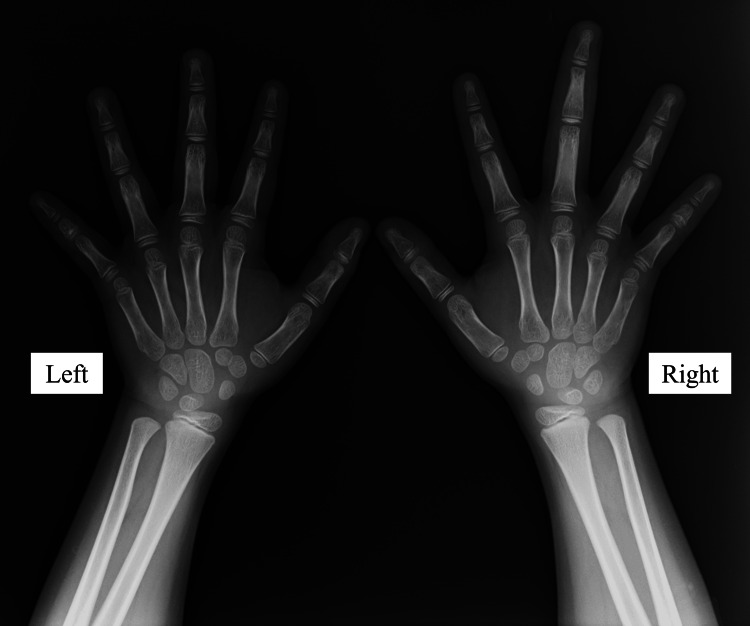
Radiographic images of the patient’s finger at the first visit. The phalanx of the middle finger is longer than that of the other fingers.
